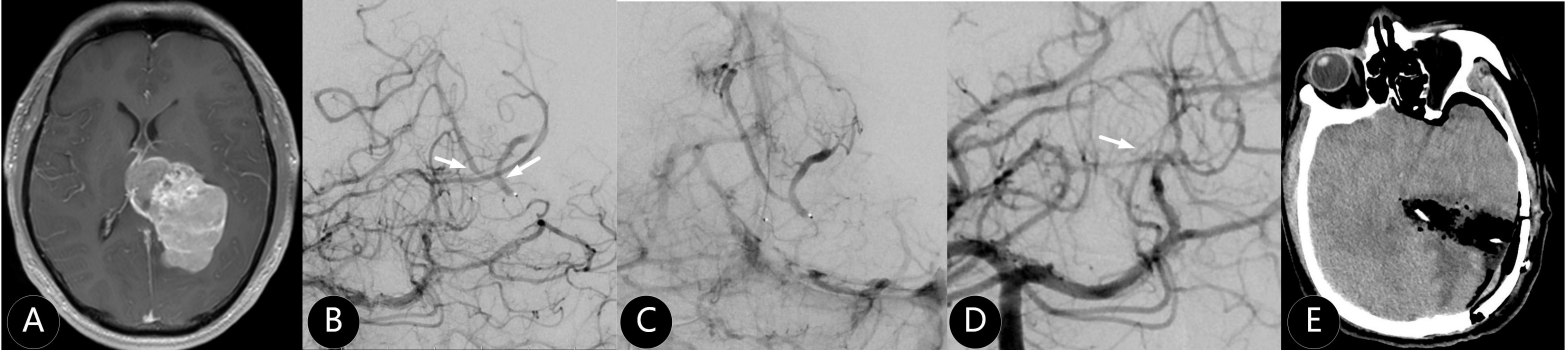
The patient suffered from severe headache for two weeks. (A) Enhanced MR imaging showed left paraventricular tumor with 120 cm^3^. (B, C) The branches of posterior cerebral artery and superior cerebellar artery were the main supplying arteries. (D) One supplying artery was embolized (white arrow) by Onyx 18. (E) The meningioma was completely removed and WHO III grade was confirmed by pathology.
